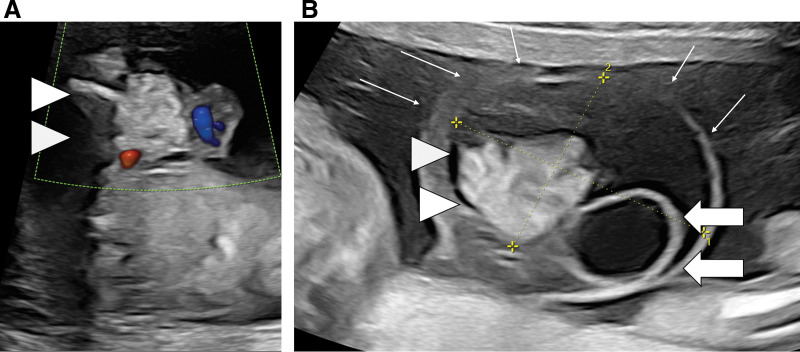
Fetal ultrasound findings. (A) Fetal ultrasound performed at 16 weeks 3 days gestation, showing an intestinal prolapse within the umbilical cord (arrowhead). (B) Fetal ultrasound at 20 weeks 3 days gestation, showing an omphalocele measuring 48 × 34 mm (thin arrow), an intestinal prolapse (arrowhead), and a large umbilical cyst measuring 18 × 16 mm (arrow).
